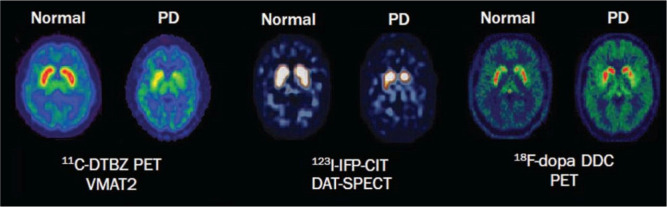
Molecular imaging of dopaminergic dysfunction in Parkinson's disease (PD) involves the use of PET (positron emission tomography) and SPECT (single-photon emission computed tomography) imaging techniques. In PD patients, these imaging studies reveal a reduction in VMAT2 (type 2 vesicular monoamine transporter) activity, DAT (dopamine transporter) availability, and DDC (dopa decarboxylase) activity when compared to healthy individuals used as controls. PET and SPECT imaging provides valuable insights into the molecular changes associated with PD, showcasing lower levels of VMAT2, DAT, and DDC functions, which are indicative of dopaminergic dysfunction in the disease [48].
